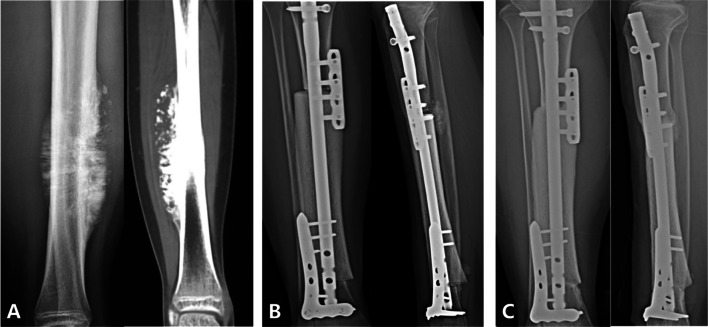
A 14-year-old female with osteosarcoma of the right tibia. A X-ray and CT scan showed tumor was located in the right tibial diaphysis. B The allograft bone was implanted and reconstructed with intramedullary nailing and two short plates. C At 25 months postoperatively, the proximal and distal osteotomy sites had excellent consolidation
